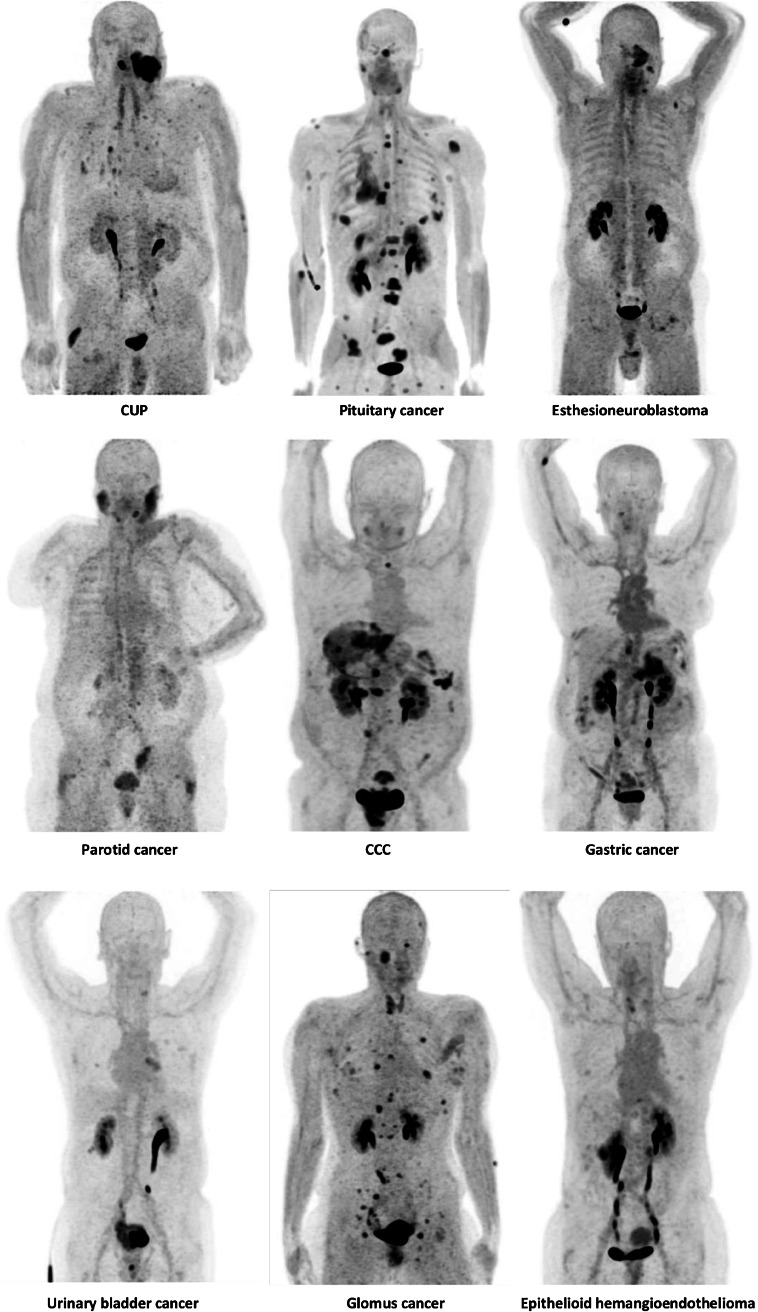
Maximum-intensity projections of ^68^ Ga-FAPI-PET/CT reflecting 9 different rare malignancies
